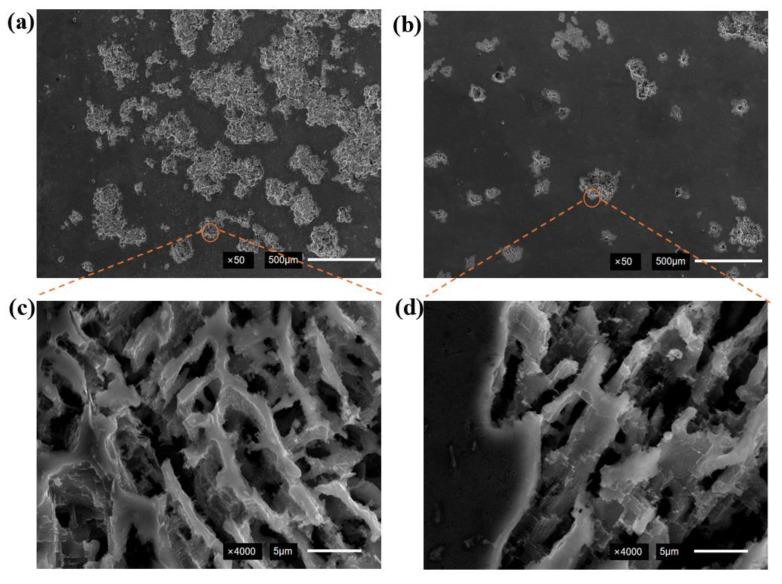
Corrosion morphology of SEM: (a) no ultrasonic; (b) ultrasonic; (c) no ultrasonic; (d) ultrasonic.
